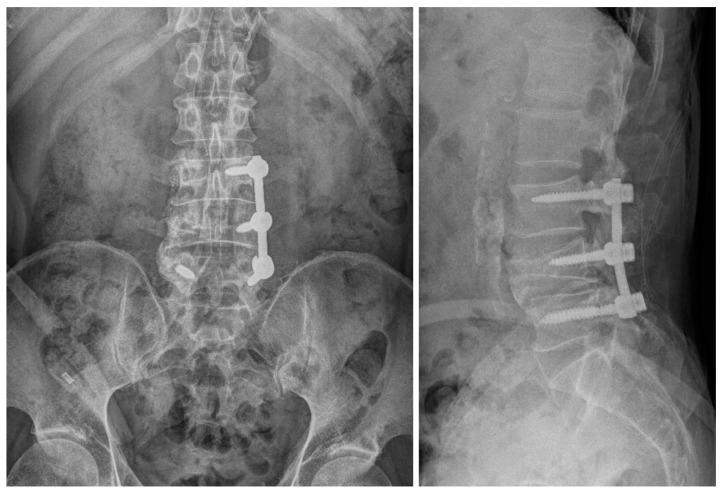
Radiographic images after the third surgery showing a well-decompressed state.
